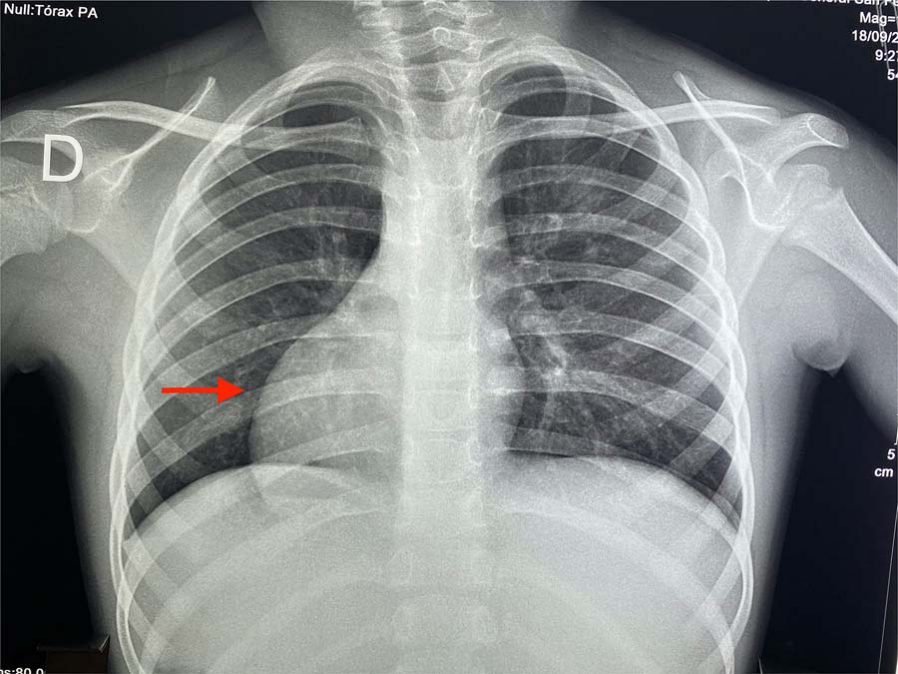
Chest radiograph. Consistent with dextrocardia. (red arrow).
